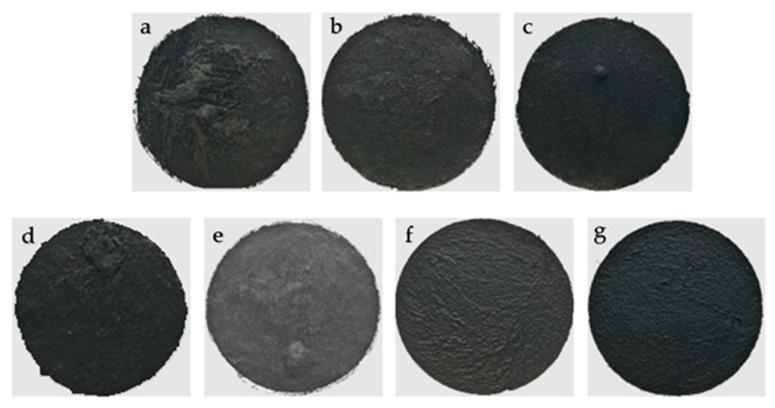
Photographs of CCGA prepared via various ultrasonic conditions of (a) 600 W, 40 min; (b) 800 W, 40 min; (c) 1000 W, 40 min; (d) 1000 W, 10 min; (e) 1000 W, 30 min; (f) 1000 W, 50 min; (g) 1000 W, 70 min.
